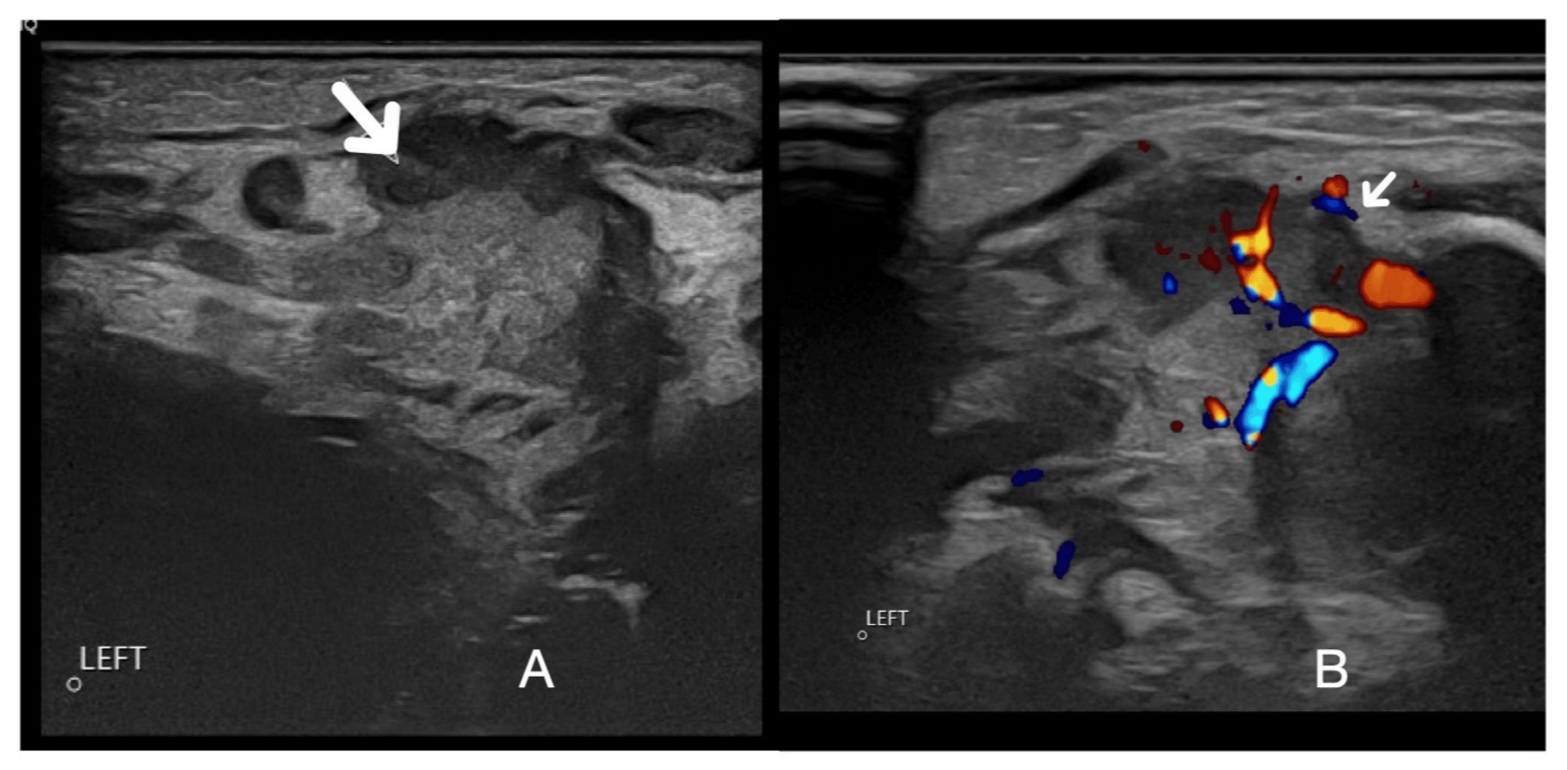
Repeat ultrasound scan of the left submandibular gland. (A) Left submandibular gland showing diffuse enlargement and heterogeneity (arrow). (B) Hyperaemia of the gland (arrow)  and continued surrounding soft-tissue inflammation.
